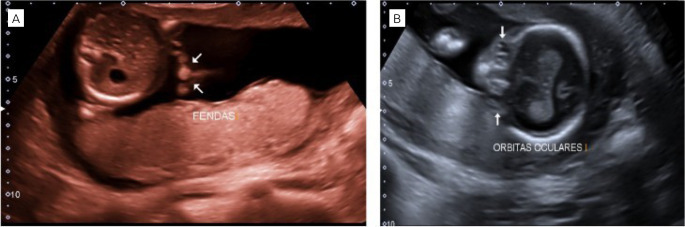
Obstetric ultrasound in Patau syndrome showing: (2A). Complex upper lip cleft; (2B). Hypotelorism with calcification in the eyeball;
